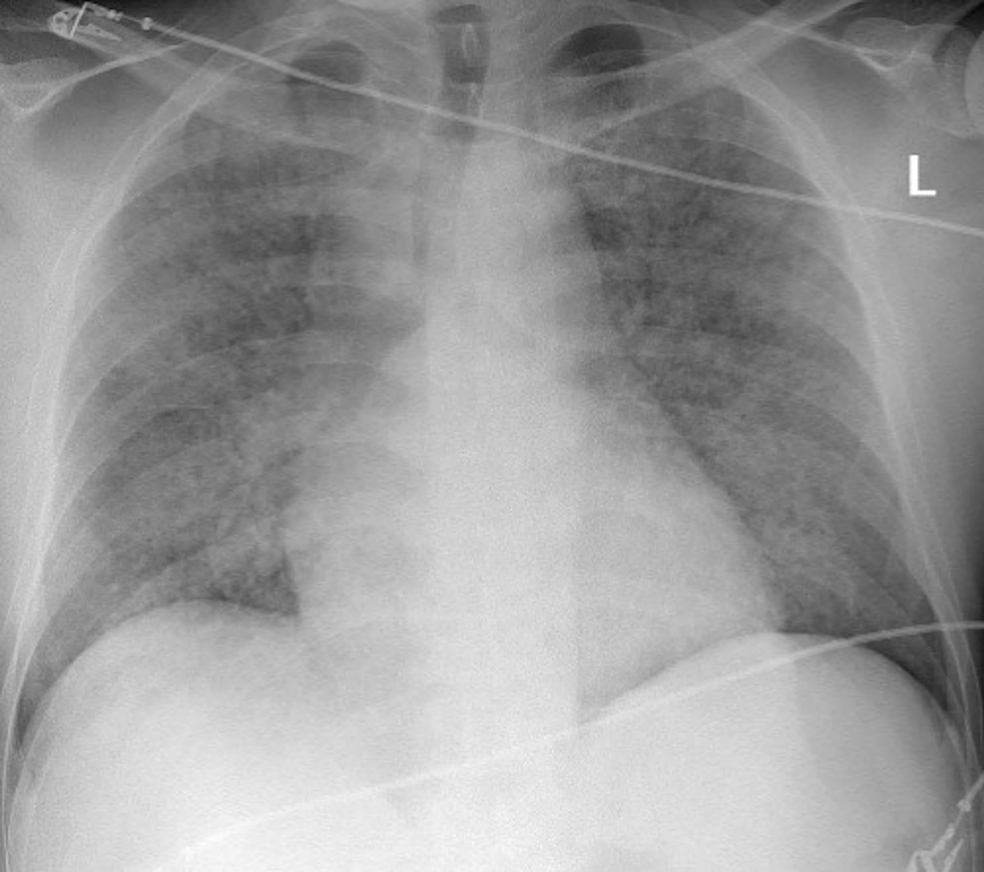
Anteroposterior chest X-ray performed at admission showing bilateral infiltrations suggesting diffuse alveolar hemorrhage
